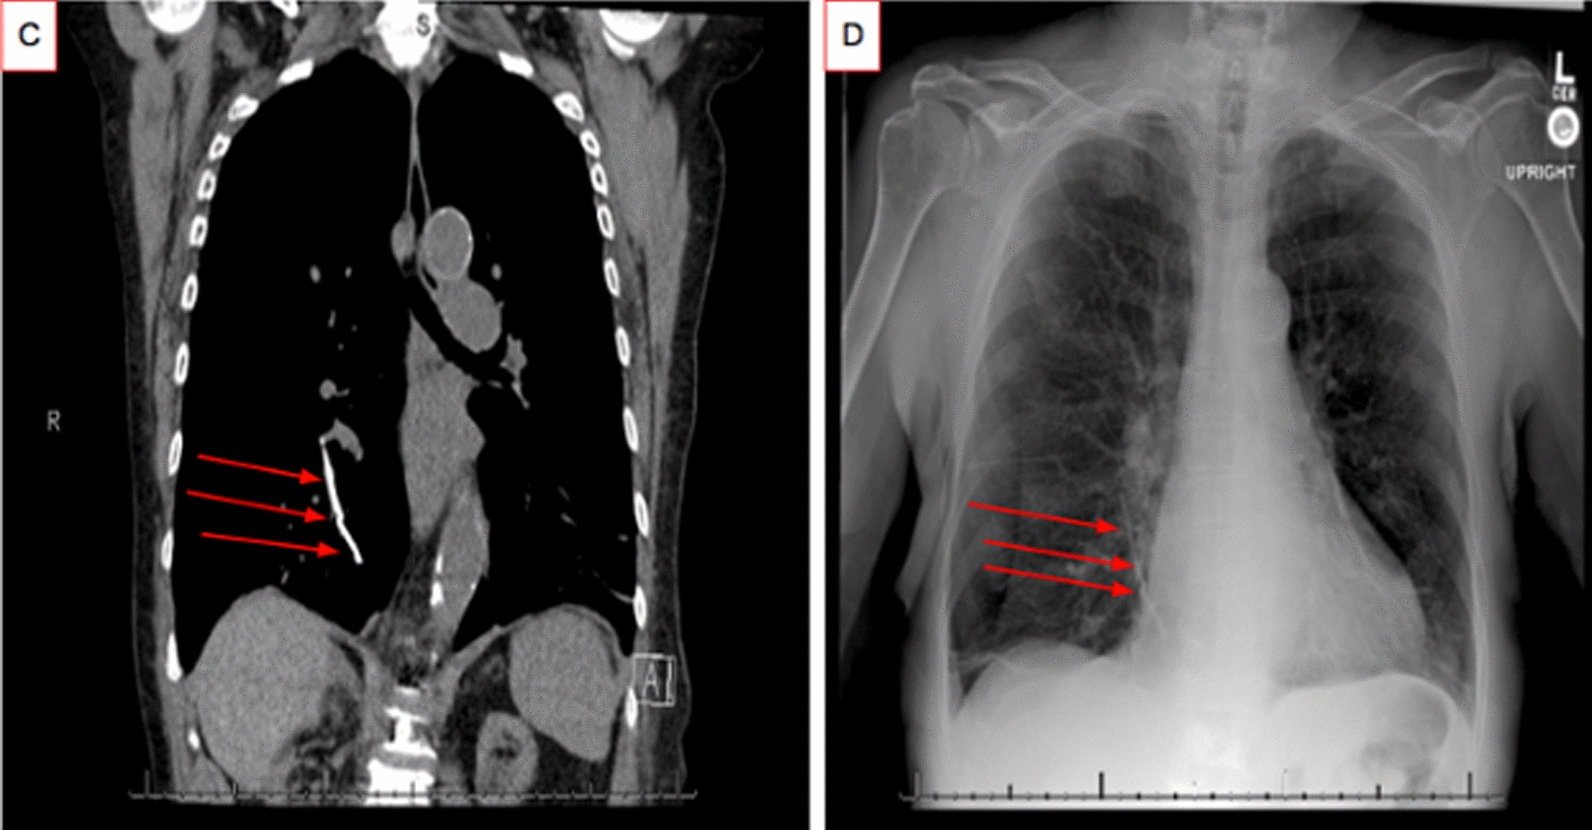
Readmission images demonstrating intravasation of the cement. CT imaging (C) and chest x-ray (D) showing cement in the right lower lobe
